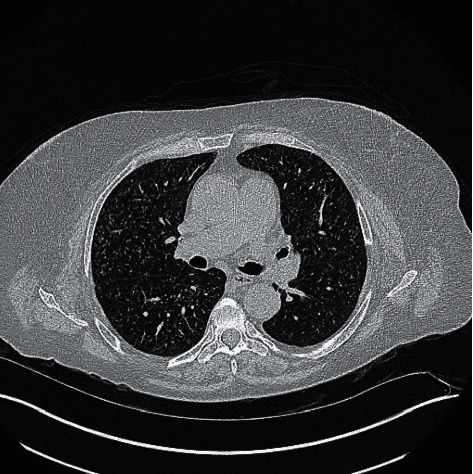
CT scan axial view showing conforming military tuberculosis with numerous 1–3 mm punctuate nodules.
